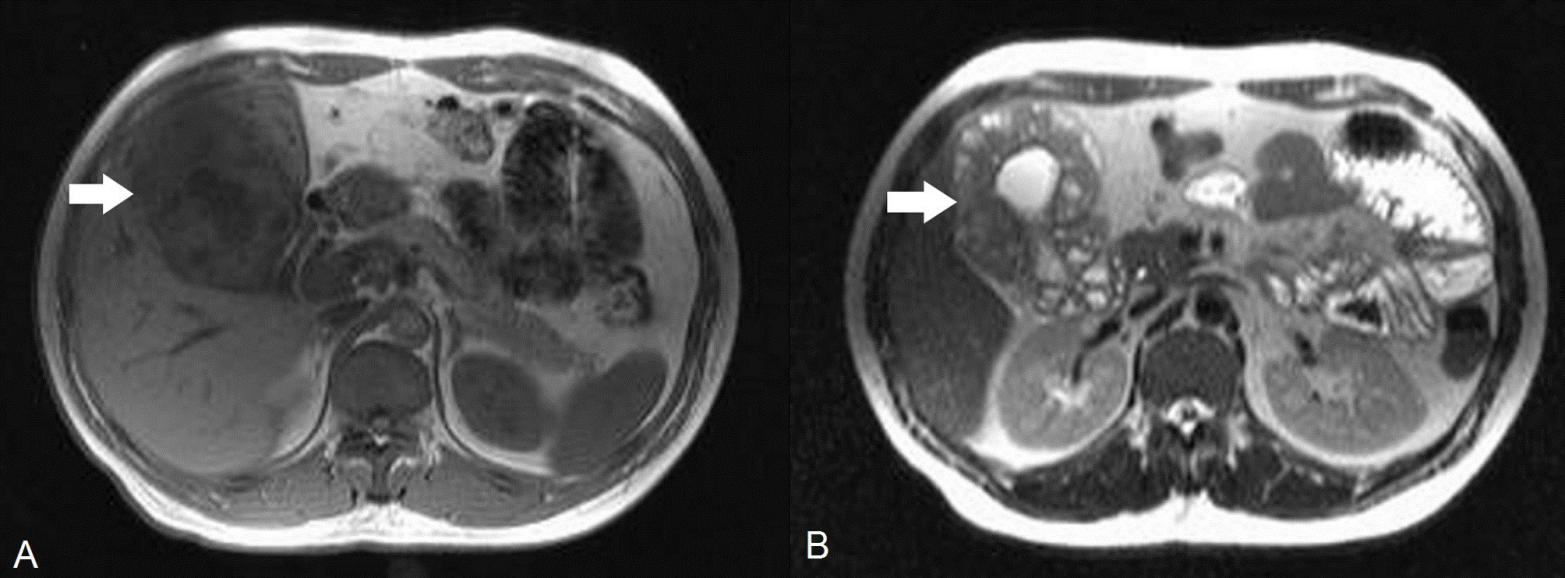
Magnetic resonance imaging of the abdomen. Marked thickening of the epithelial and muscular elements in the gallbladder with multiple variably sized intramural cysts (arrow) was observed in the T1- (A) and T2-weighted (B) sequences, which is consistent with adenomyomatosis of the gallbladder with intramural Rokitansky-Aschoff sinuses.
